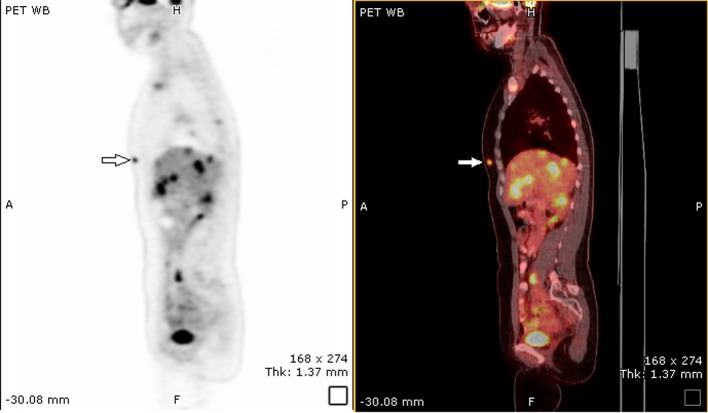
PET scan showing FDG uptake in right breast and liver.
